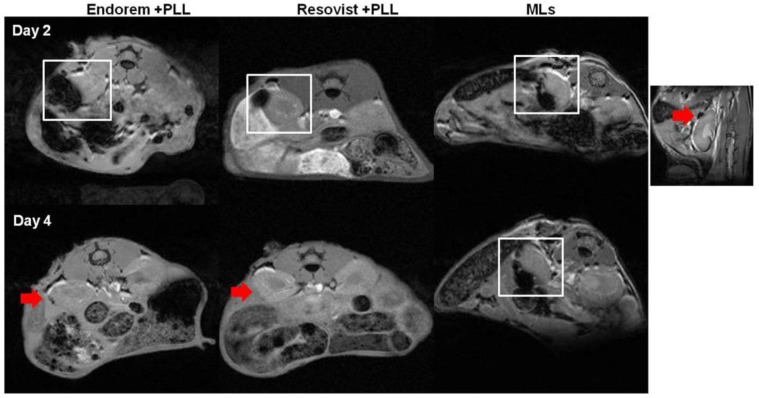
MR imaging of transplanted islets: MR imaging was performed to detect the labeled islets in vivo. The hypointense sub-capsular region (highlighted by white square) indicates PIs labeled with Endorem + PLL and Resovist + PLL. Endorem or Resovist + PLL-labeled islets were not detectable on day four in the sub-capsular region (red arrows), whereas PIs labeled with MLs were detectable until day four (indicated by white square). The right corner image indicates a prominent black spot (red arrow) seen on the sub-capsular region after transplantation of PIs labeled with MLs.
